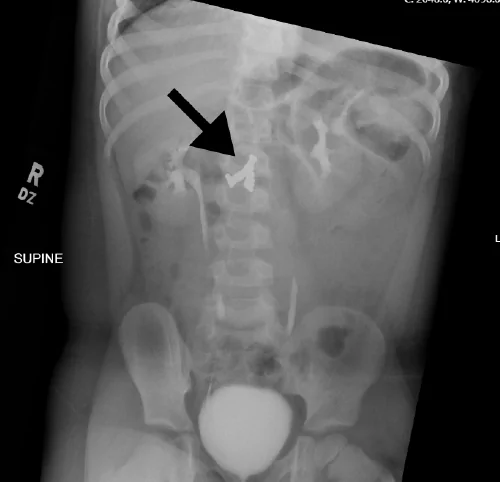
Radiograph demonstrating ingested stacked magnets (arrow).
